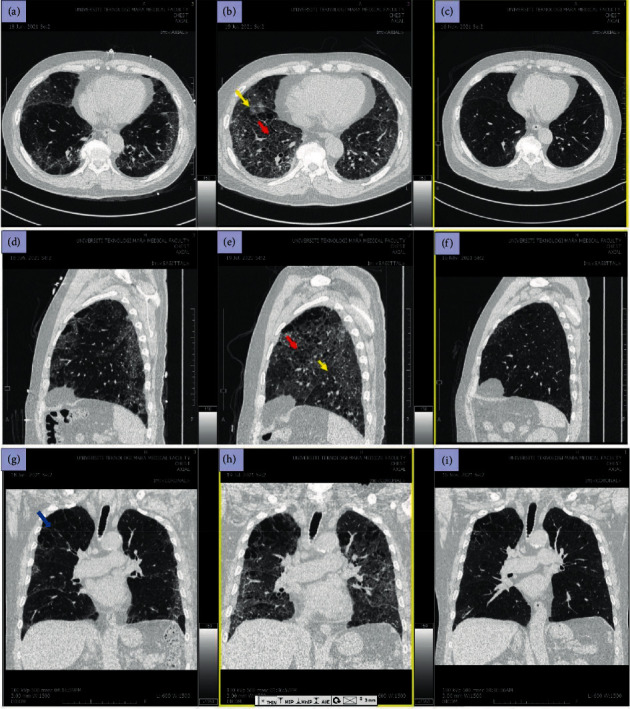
The sequences of CT thorax at day 10 of COVID-19 illness (a–c) showed moderate GGO with organizing pneumonia in the background of emphysematous lung (blue arrow). Second CT thorax at day 42 post COVID-19 infection (d–f) showed worsening bilateral ground glass opacity with reticulations and crazy paving pattern (red arrow) most prominent at bilateral lower lobes. There is also reduction in lung volume with displaced fissures (yellow arrow). Third CT thorax at 5 months post COVID-19 infection (g–i) showed significant improvement of GGO with residual reticulations in apical region and lung bases.
